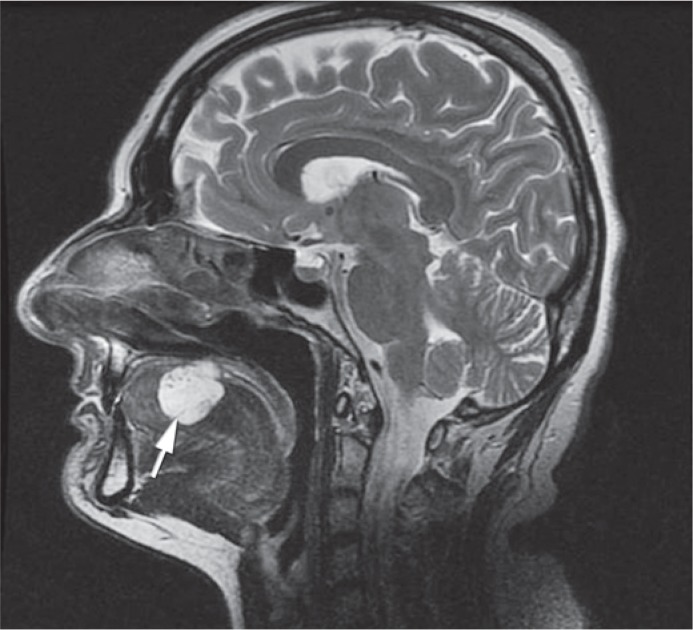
T2 weighted MR image in sagital plane before treatment shows 35 × 25 mm big lesion (white arrow) identified as submucosal cavernous haemagioma of the tongue. The lesion is expansive and does not involve the radix of the tongue.
